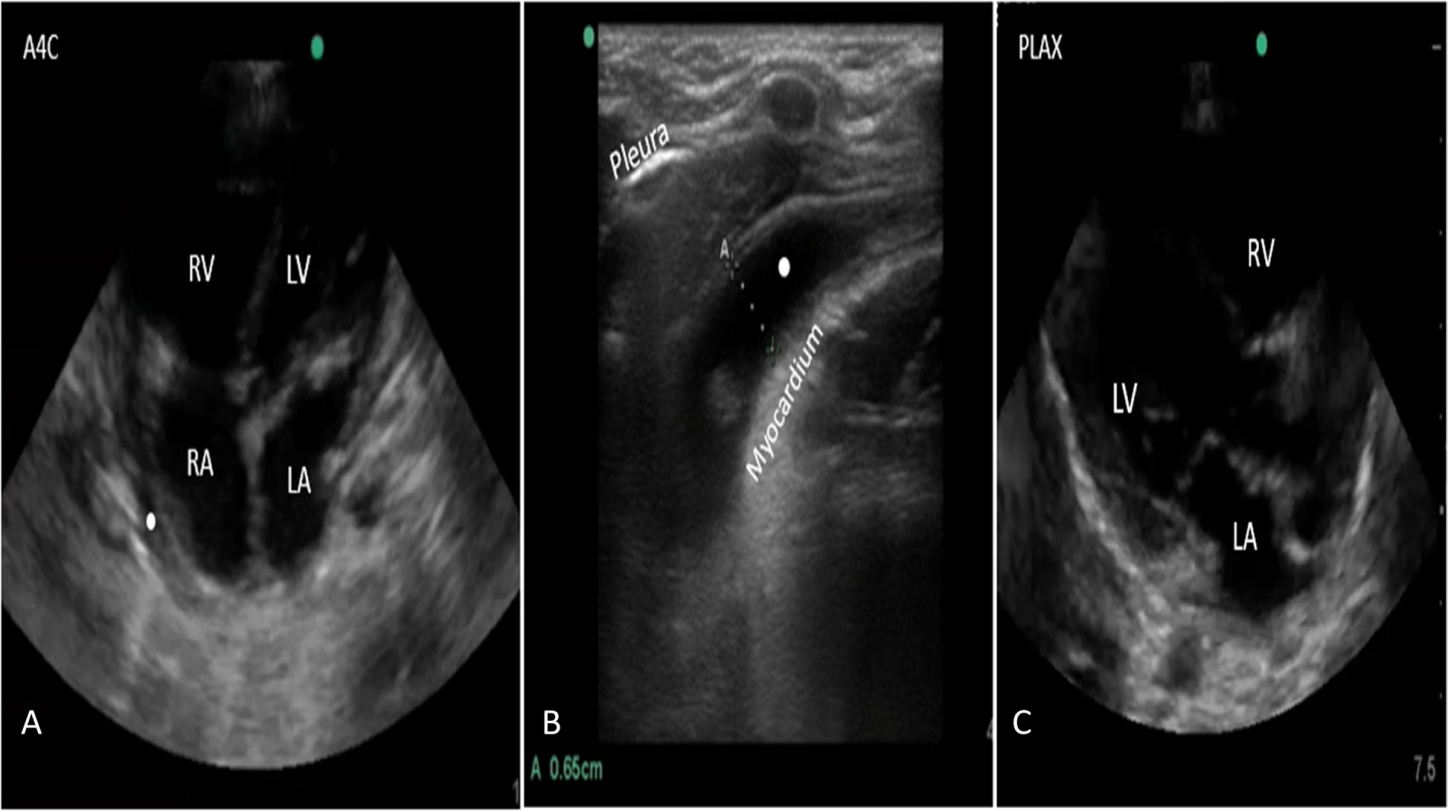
Cardiac ultrasonographic views following the procedure. A Apical four-chamber (A4C) view showing a decrease in the pericardial effusion (white dot). B Right parasternal view (longitudinal view axis) demonstrating a reduction in diameter of the cardiac effusion (white dot).The patient was referred to a specific pediatric facility and then diagnosed with tuberculosis. C Echocardiography scan and parasternal long-axis view revealing full clearance in pericardial effusion at 2 weeks after pericardiocentesis
